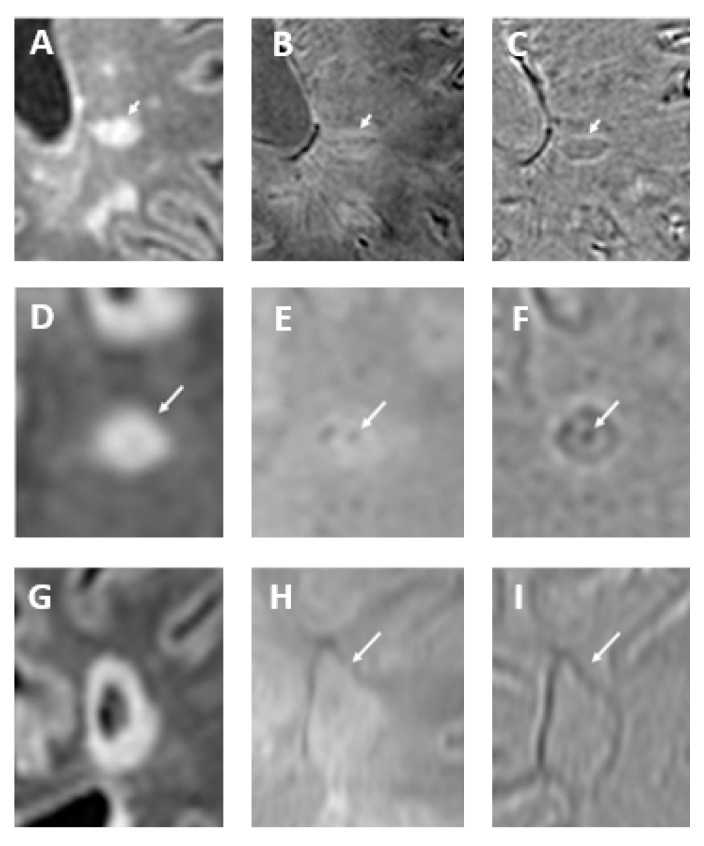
In oval-shaped lesions on fluid-attenuated inversion recovery (FLAIR) sequence (arrow in (A)), the central vein is visible as a hypointense line on SWI (arrow in (B)) and SWI-phase (arrow in (C)), creating a coffee-bean appearance. Conversely, in round-shaped lesions on FLAIR image (arrow in (D)), the central vein appears as a dark point on SWI (arrow in (E)) and frequently forms a target or doughnut shape on SWI-phase (arrow in (F)). Paramagnetic rim lesions are visible in both SWI and SWI-phase images. The FLAIR image (G) depicts a cavitated multiple sclerosis plaque, with a paramagnetic rim highlighted in both the SWI (arrow in (H)) and SWI-phase (arrow in (I)) images. Modified from Rimkus et al. [18] under the terms and conditions of the Creative Commons Attribution (CC BY) 4.0 license (https://creativecommons.org/licenses/by/4.0/ (accessed on 1 March 2025)).
